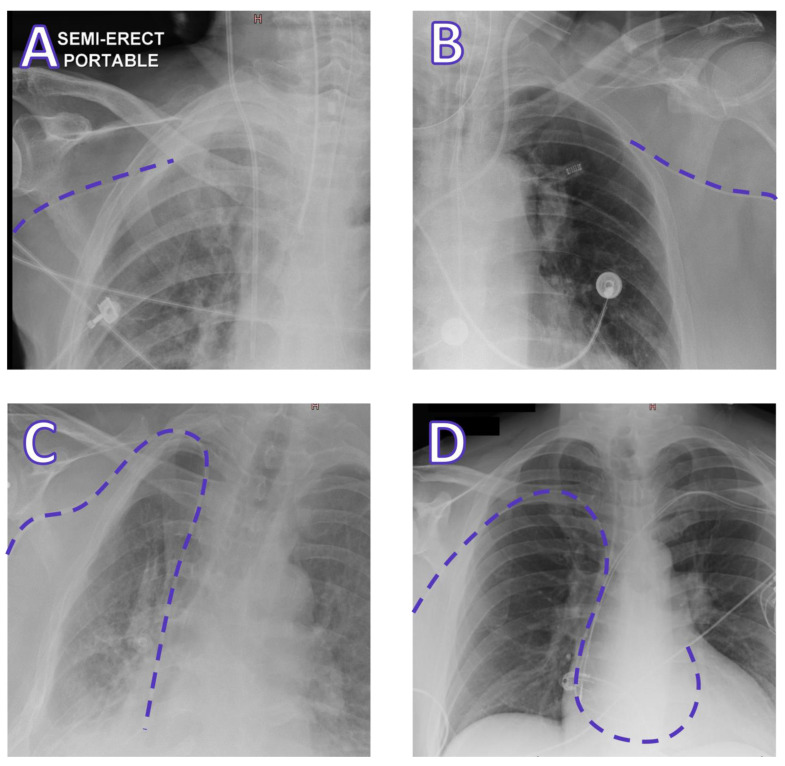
Representative examples of false negative malpositioned PICCs, with paths highlighted by dashed lines: (A) A right-sided PICC terminating in the subclavian vein; (B) A left-sided PICC terminating in the subclavian vein; (C) A right-sided PICC with the tip in the proximal right atrium; and (D) A brachial Swan-Ganz catheter with tip in the right-ventricular outflow tract. These are some examples of malpositioned lines that were not classified by the model as being malpositioned. Cases are from a test dataset containing radiographs from a private Australian radiology practice and the MIMIC-CXR dataset [35].
